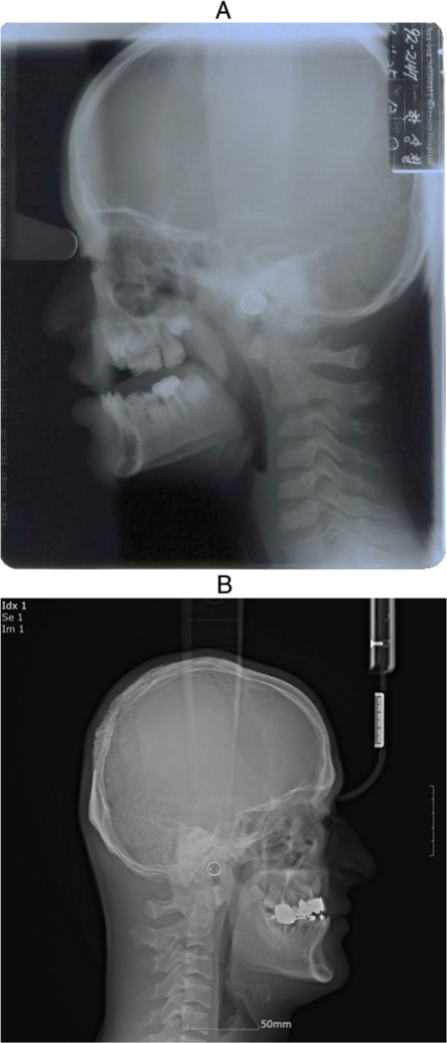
Lat cephalometric radiographs at the age of 11 and 29 show the tendency of maxillary hypoplasia and pseudo-mandibular prognathism due to midface deficiency. a At the age of 11. b At the age of 29
